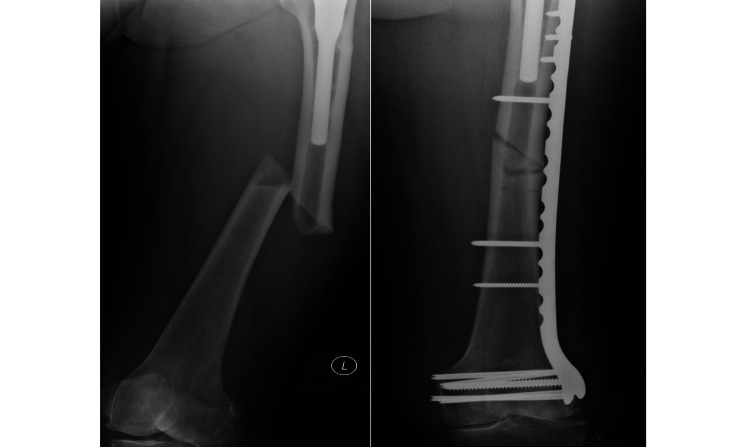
Before and after radiographs for a patient managed with plate fixation (which subsequently failed due to non-union)
